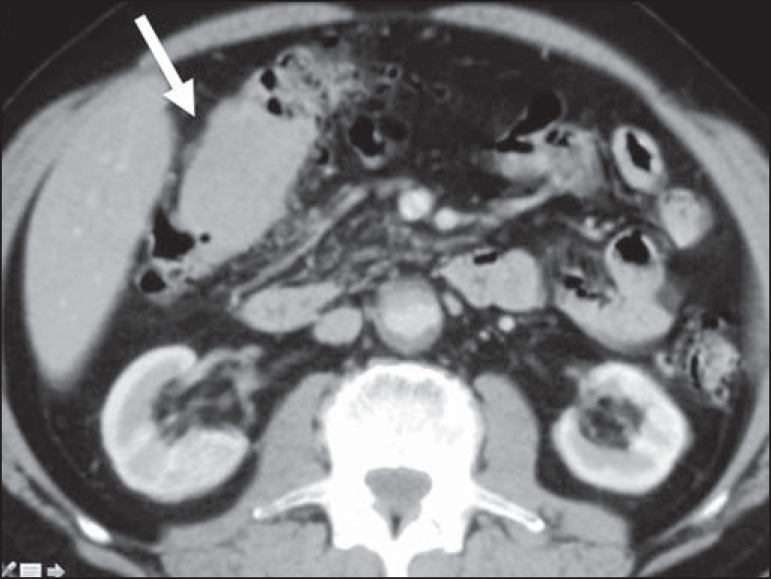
Lymphoma of the colon. Contrast-enhanced axial CT of the abdomen, showing
